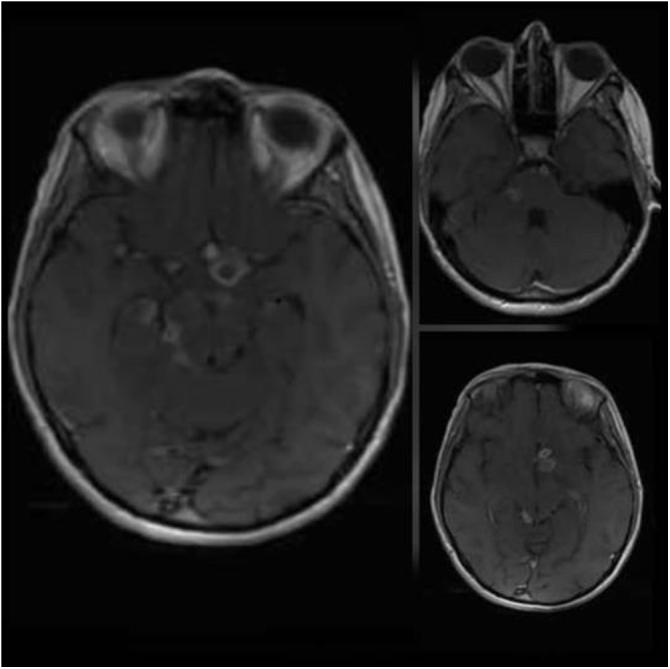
Axial T1-weighted MRI brain post-gadolinium demonstrating multiple ring-enhancing lesions.
